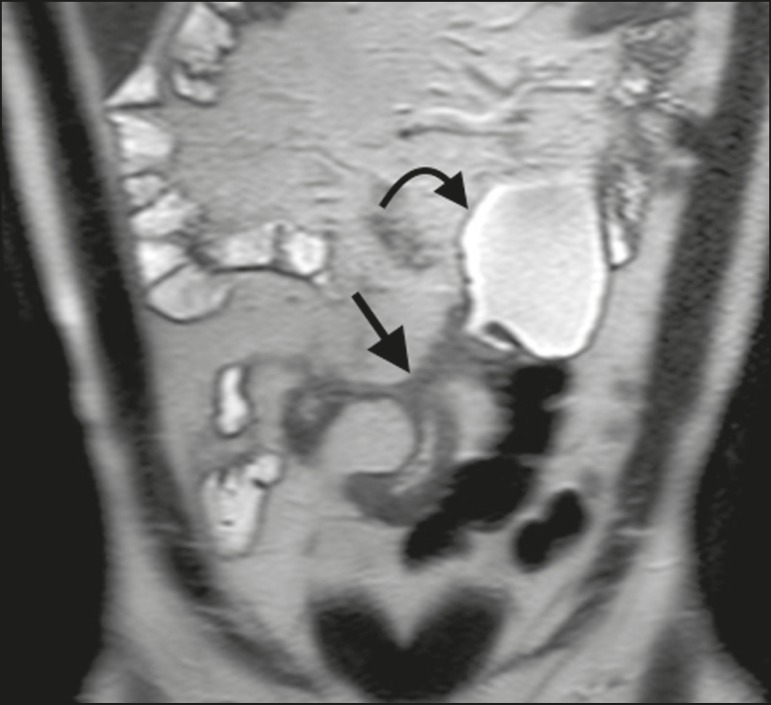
Coronal T2-weighted MRE sequence showing stenosis and enteroenteric fistula in the distal ileum, with the “clover-like” sign (straight arrow). Note also the upstream dilatation of the prestenotic segment (curved arrow).
